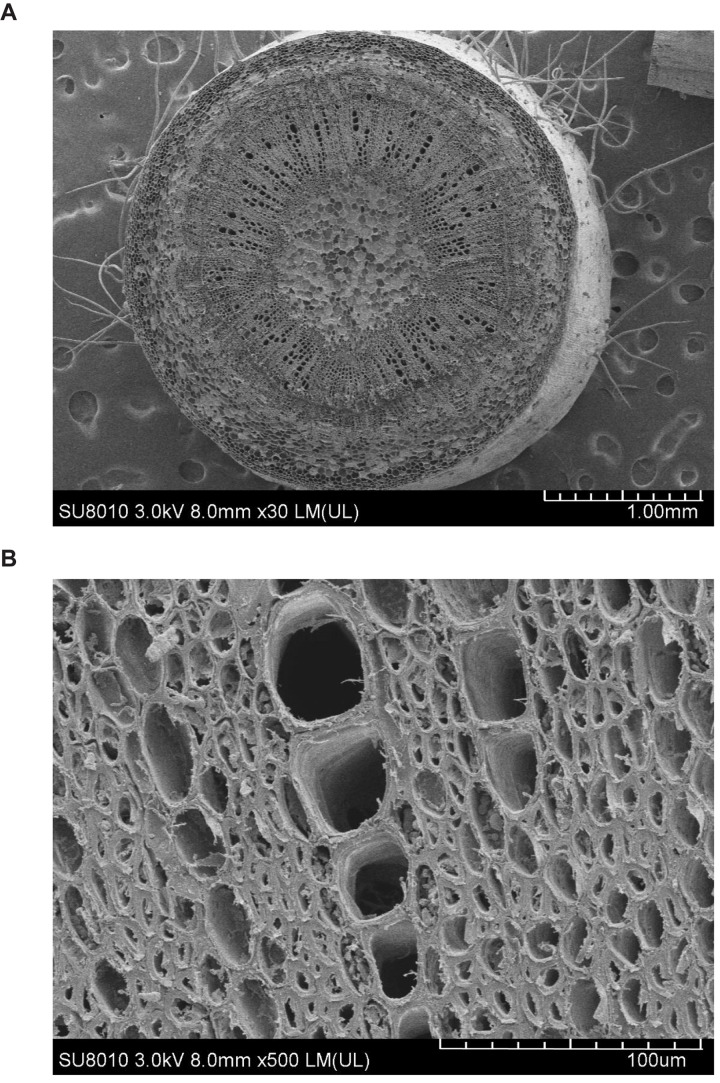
SEMs of the Ji863 cotton cultivar at two contrasting scales show the xylem vessels which are comparable to those captured in the 3D CT image in Figure 1. (A) SEM of the stem, (B) SEM to highlight the xylem vessels.
